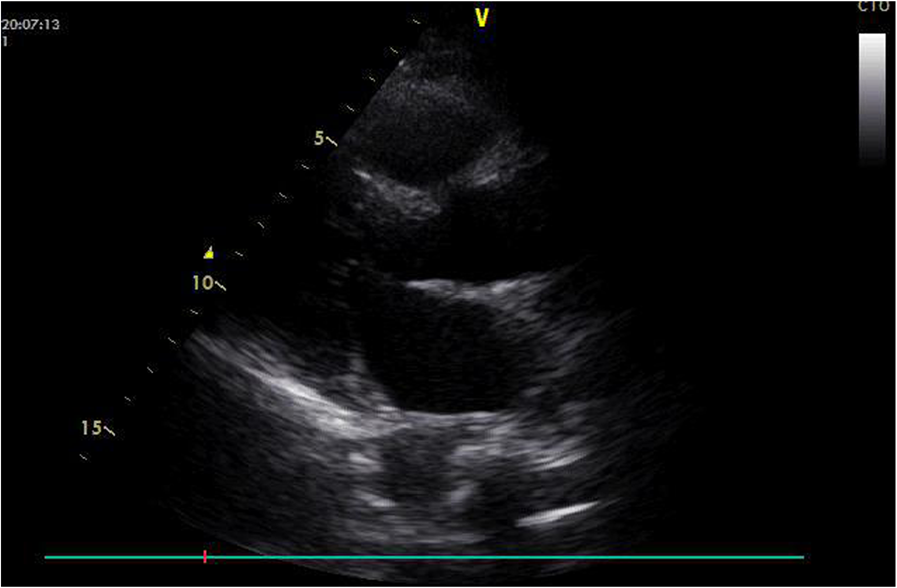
Trans thoracic echocardiography(TTE) just after ventricular fibrillation. TTE showed no air bubble in the heart
